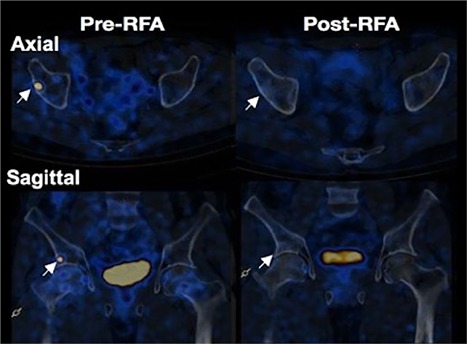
Pre‐ and post‐RFA Ga^68^ DOTANOC PET/CT scans of patient 1. Axial and sagittal views of the lesion in patient 1. Post‐RFA images demonstrate disappearance of the uptake at the lesion site. Arrows indicate the lesion.
